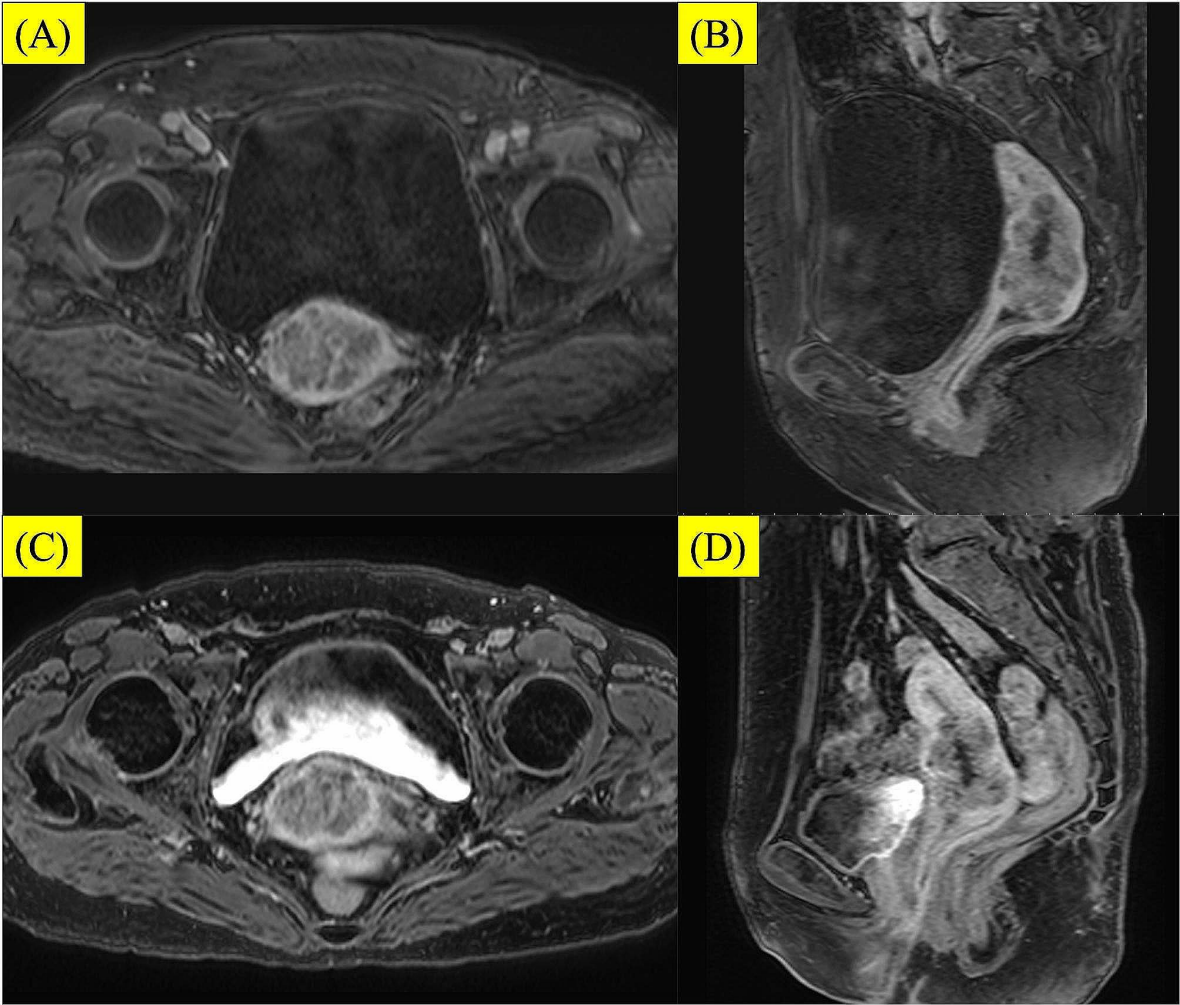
A, B) Pretreatment contrast-enhanced magnetic resonance imaging (MRI) showed a bulky tumor in the cervix. C, D) Interim MRI before brachytherapy showing no significant tumor shrinkage
